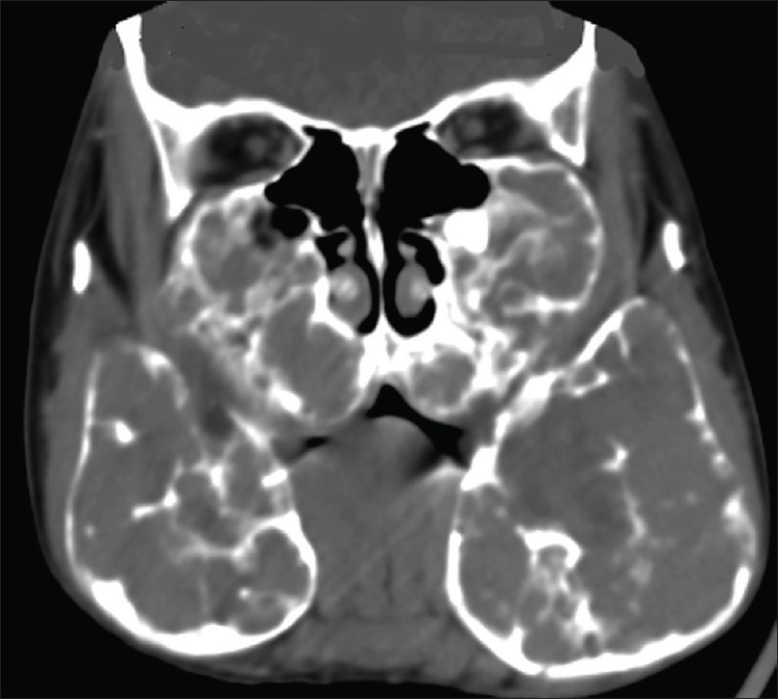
Coronal CT scan reveals expansile maxillary lesions with obliteration of the antral cavity and extension to the floor of the orbit
